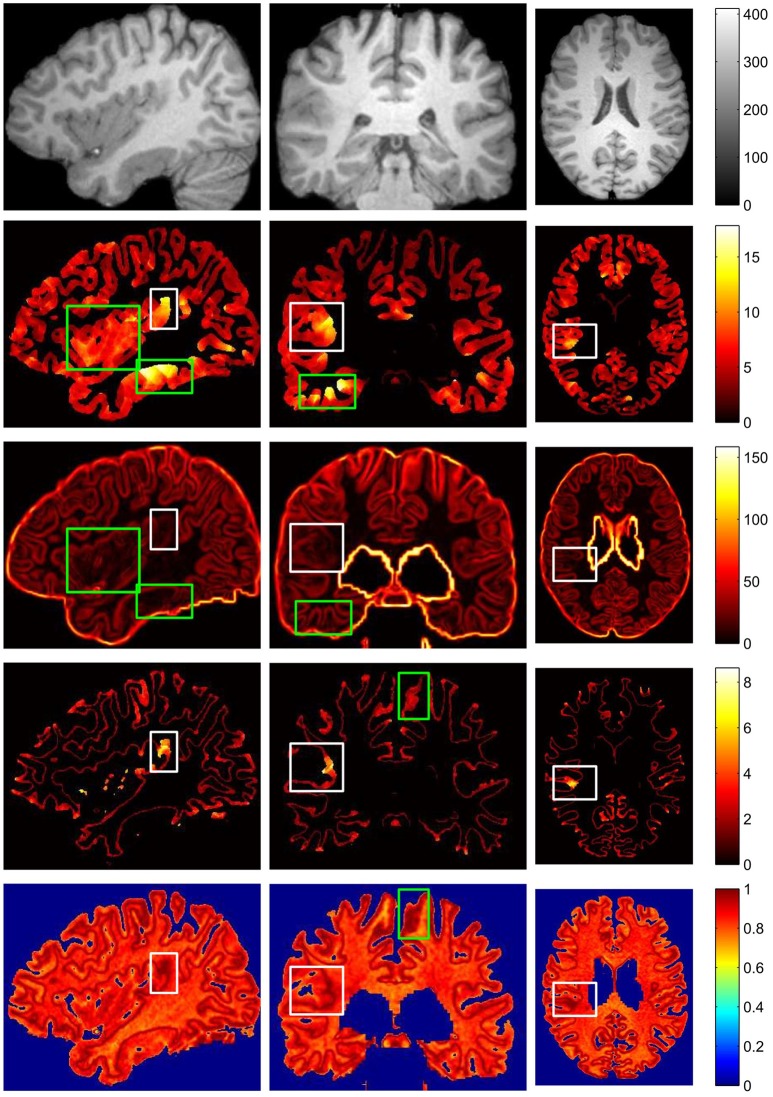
The comparison of different FCD feature maps in three views. From up to down, images on each row are preprocessed MR image, gray matter or cortical thickness map, gradient map, GWB width map, and relative intensity map. From left to right, columns are sagittal view, coronal view and axial view. The images are randomly extracted from three dimensional image from one patient. The true FCD lesional regions are surrounded by white rectangles, while the false positive regions are indicated by green rectangles.
